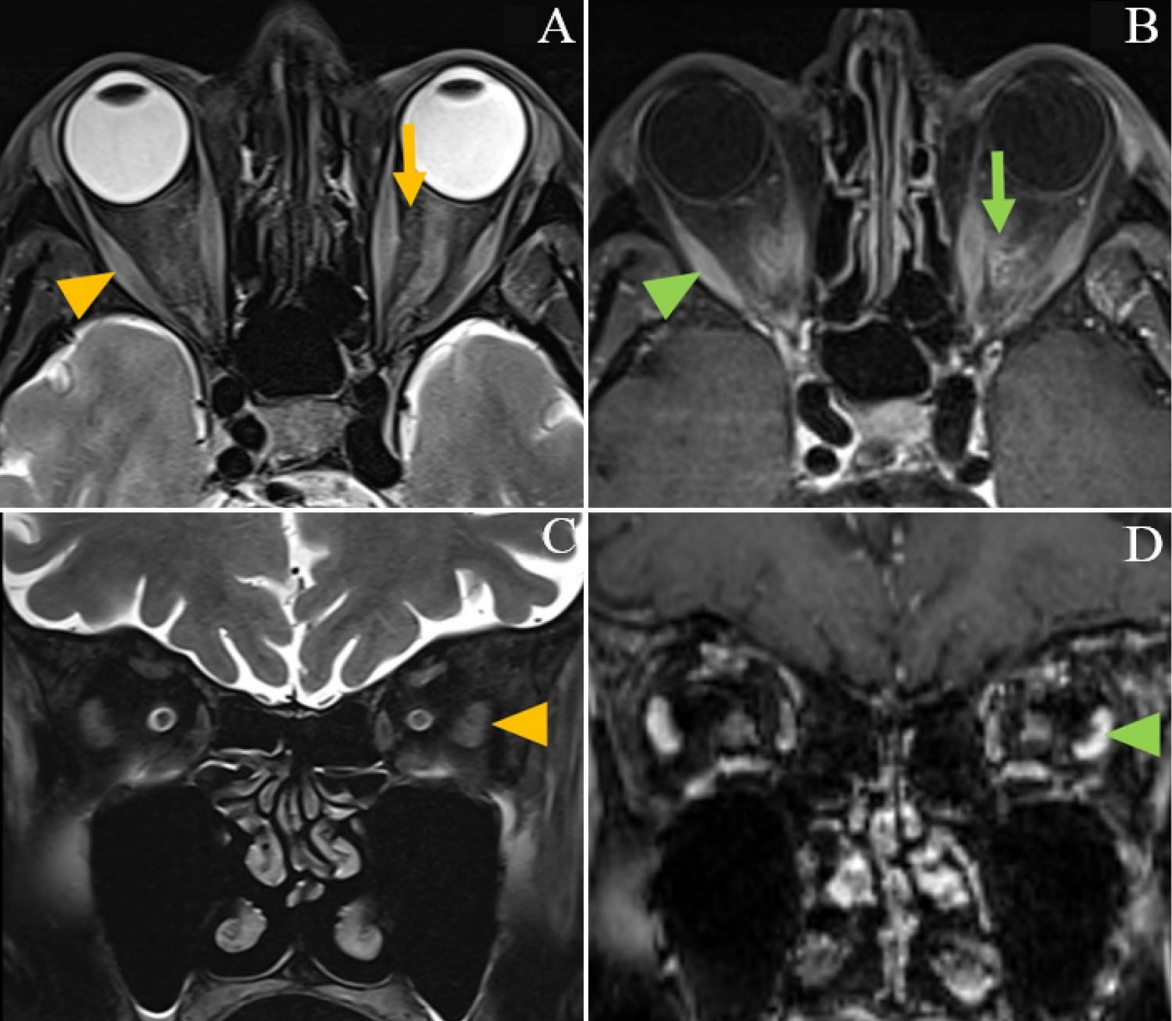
Brain MRI, patients 3 (axial view) and 1 (coronal view). Signs of orbital inflammation. In T2 fat-suppression weighted sequence (A, C), T2-hypersignal in oculomotor muscle (orange arrowheads) and intraorbital fat (orange arrow). In T1 SPACE 3D fat-saturation weighted sequence (B, D), presence of muscle (green arrowhead) and intraorbital fat (green arrow) enhancement after gadolinium injection
